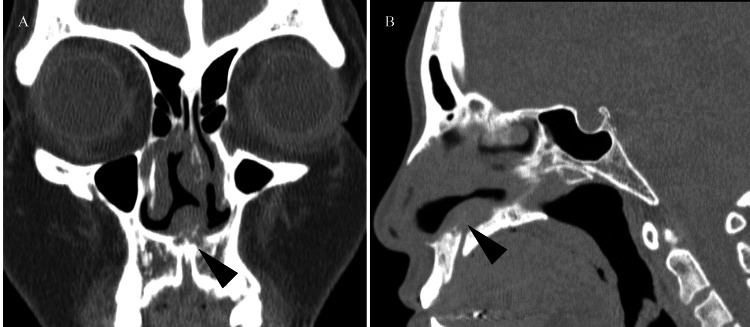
Preoperative CT scan. (A) Coronal. (B) Sagittal. Bone destruction is observed from the maxillary nasal ridge to the anterior part of the vomer (black arrowhead).
